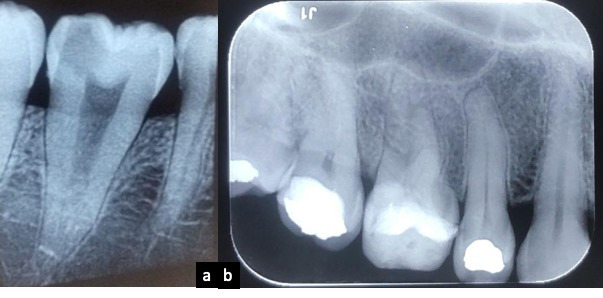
Radiografías periapicales: a) Diente 37; b) Diente 15. Se evidencia el espacio del ligamento periodontal, la lámina dura y la relación con el tejido pulpar.
